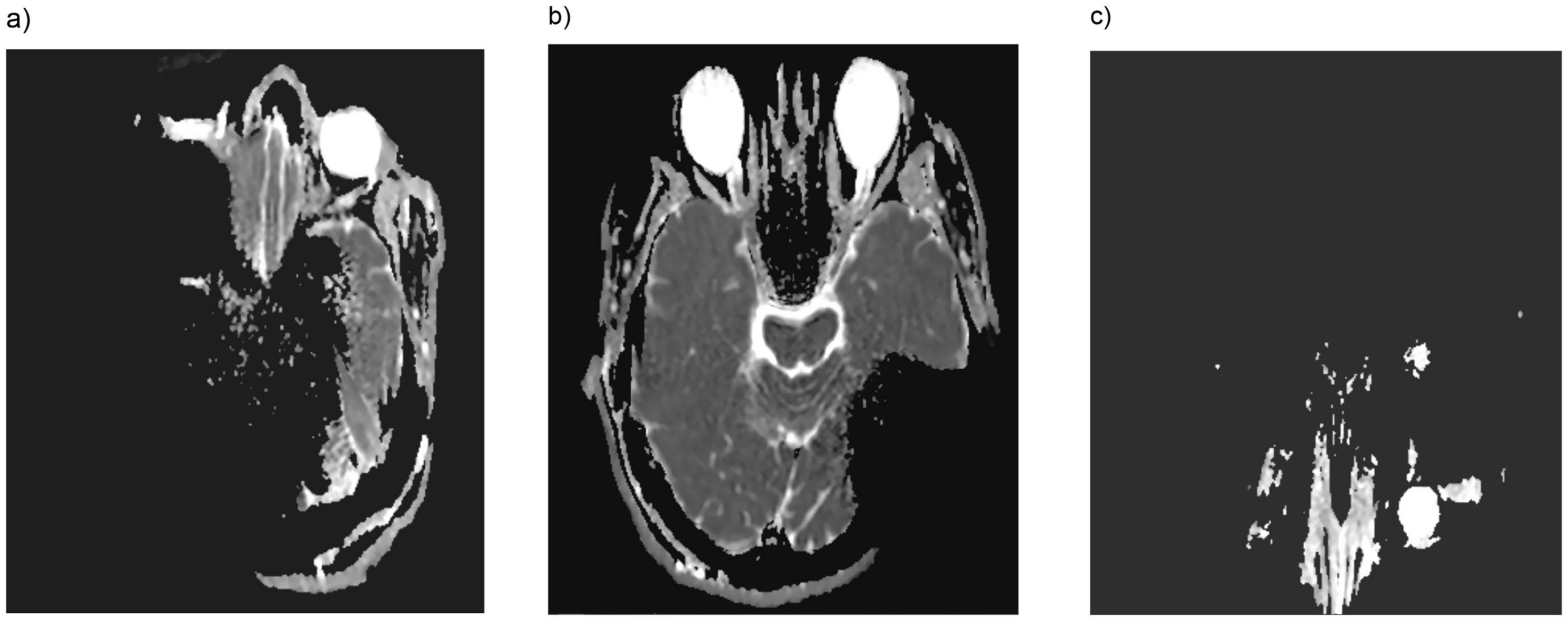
In DWI (diffusion weighted imaging) orbital accessibility for 135° cochlear implant positioning was impeded with the magnet in place unilaterally (a) or bilaterally (c), whereas without the magnet (b), the orbit was clearly visible and accessible.
